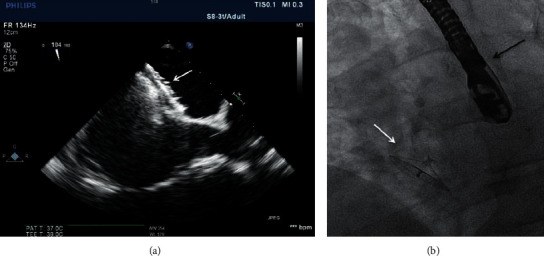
The Occlutech ASD device (white arrow) using the transesophageal echocardiography (TEE) probe (a) and fluoroscopy (b). The black arrow indicates the TEE probe during fluoroscopy.
